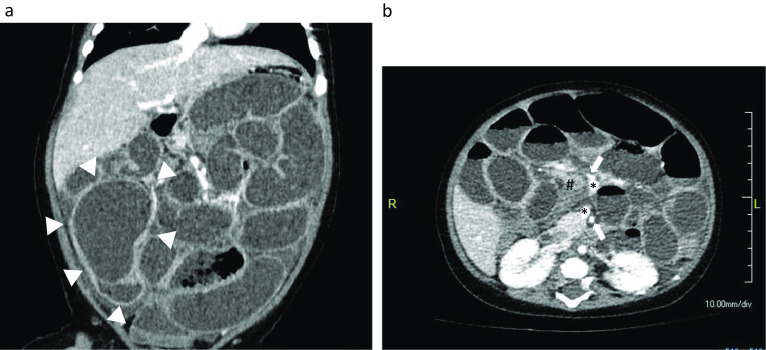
Contrast-enhanced computed tomography images of Case 1. a Cystic mass (50 mm, arrowheads) in the terminal ileum with compressed normal ileum was observed. b Stretched edematous mesentery (#) caused by twisted and dilated small intestines was observed (arrows: superior mesenteric artery; *superior mesenteric vein)
